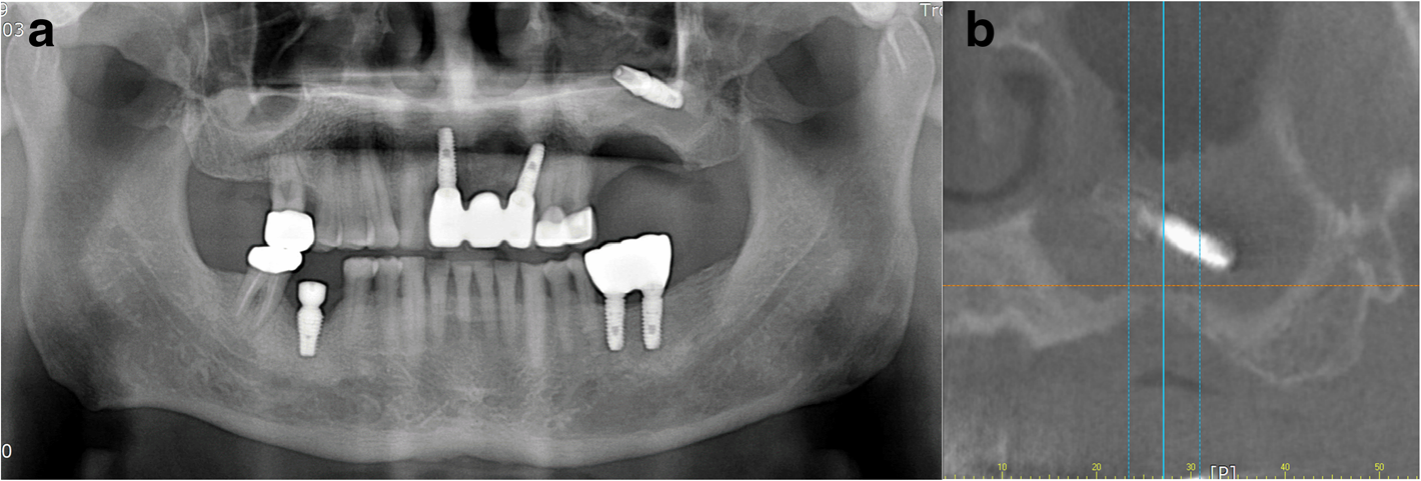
Preoperative a panoramic view and b CBCT view
